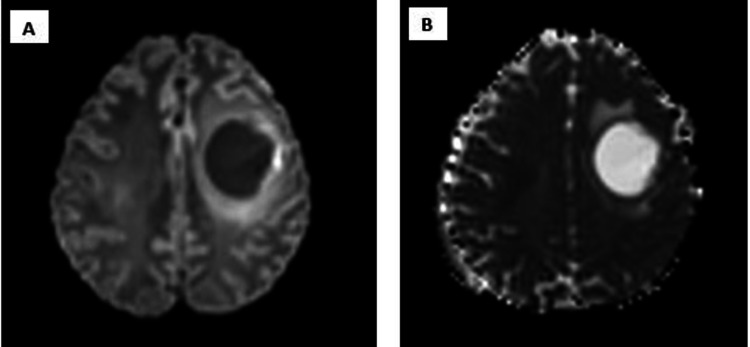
DWI/ADC sequences show restricted diffusion. DWI (A). ADC (B). DWI: diffusion-weighted imaging, ADC: apparent diffusion coefficient.
